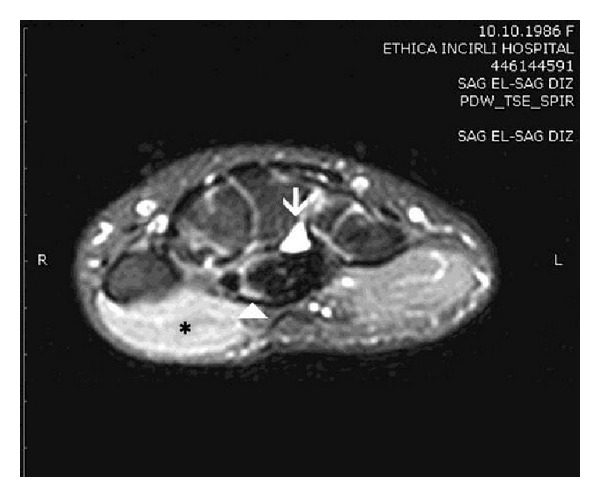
MRI revealed a space-occupying lesion (arrow) in the carpal tunnel and the median nerve (arrowhead) with high signal intensity and high signal intensity in the thenar muscles consistent with acute denervation (asterix) on fat-suppressed proton density axial images of the wrist.
